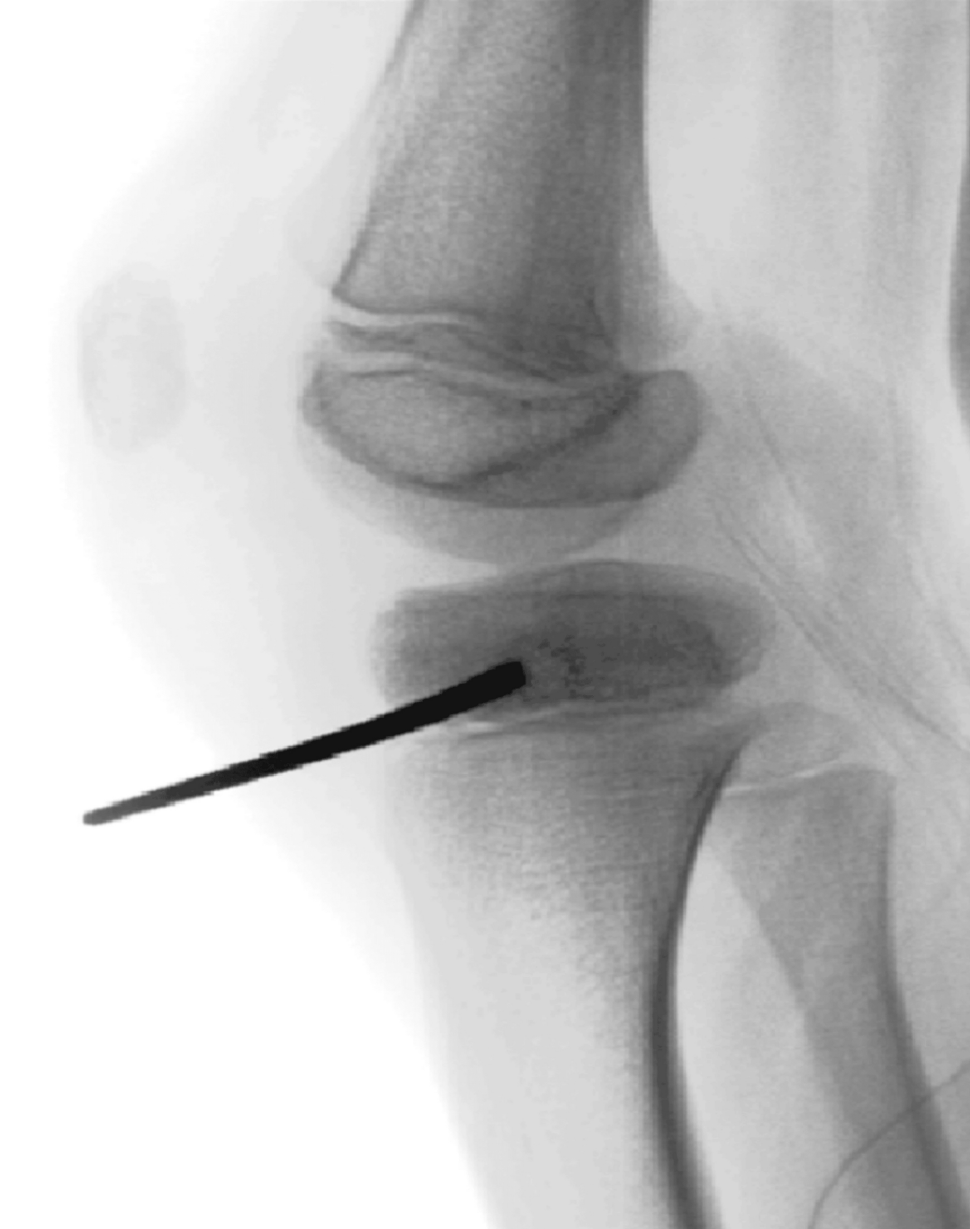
Lateral intraoperative radiograph showing the guide wire tip located at the lesion (first surgery)
